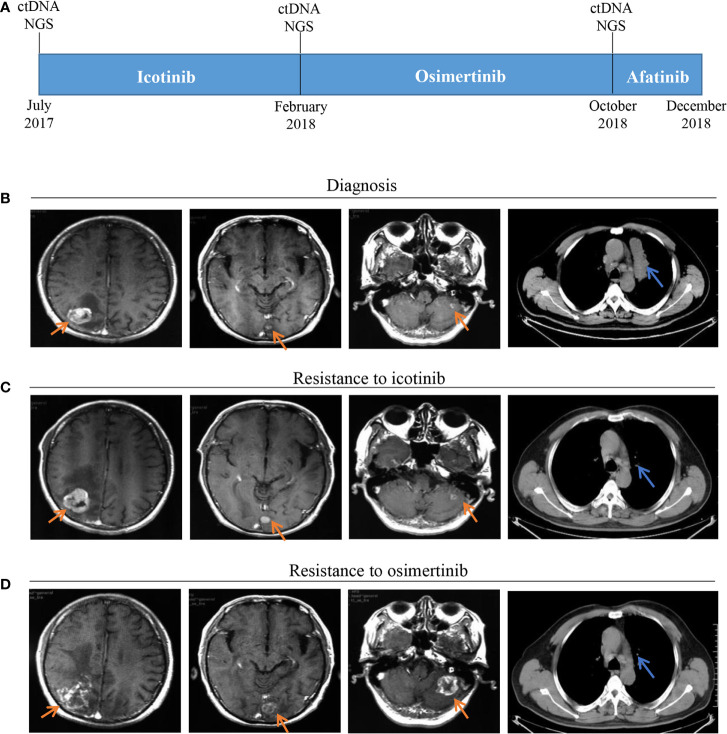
Timeline and effect of *EGFR*-TKI treatments. (A) Treatment timeline; (B) Baseline images of CT and MRI at diagnosis; (C) Brain metastases progressed and original lung mass reduced after seven months of icotinib treatment; (D) Brain metastases progressed and thoracic lesions controlled after eight months of osimertinib treatment; EGFR, epithelial growth factor receptor; TKI, tyrosine kinase inhibitor; CT, computed tomography; MRI, magnetic resonance imaging.
